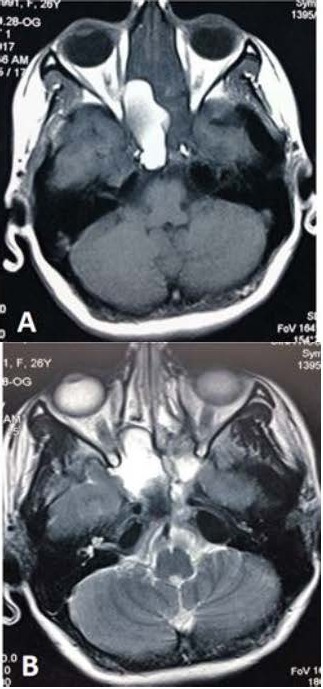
T1 (A) and T2 (B) weighted axial MRI shows a homogenous hyper-intense mass in the right sphenoid sinus, with invasion to the right optic canal and right posterior ethmoid sinus
